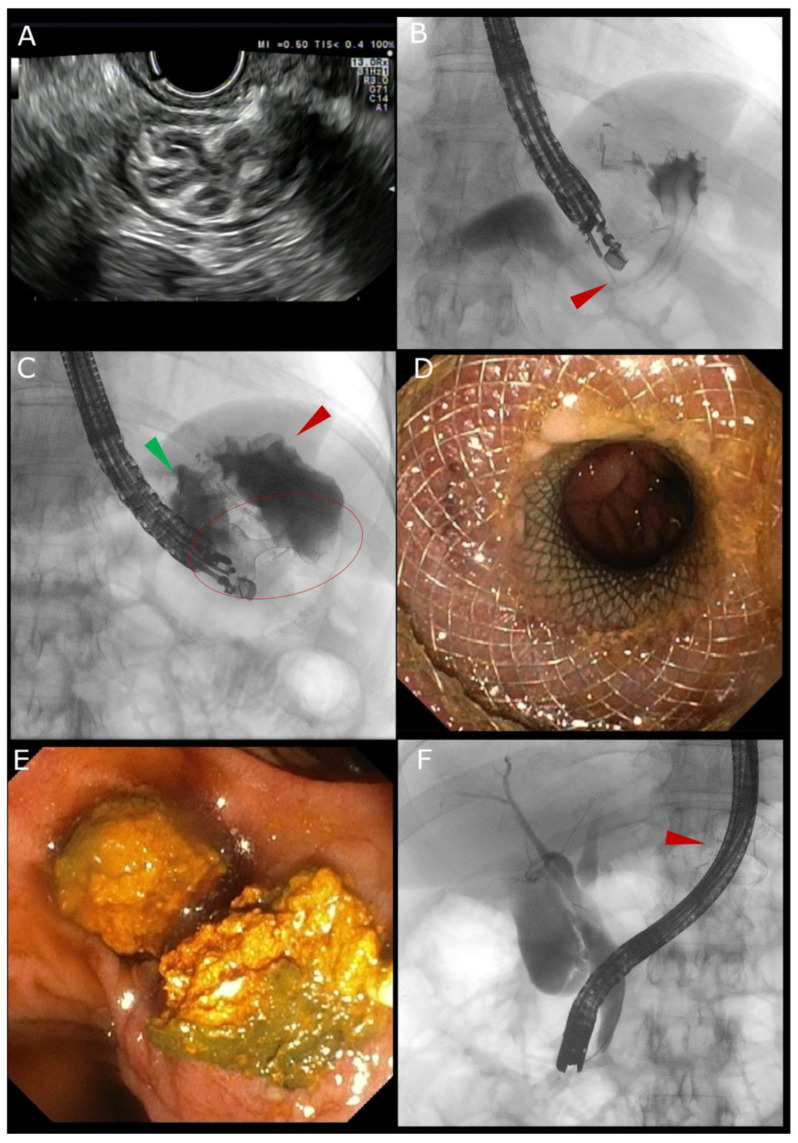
(A) Endoscopic ultrasound (EUS) view of the “sand dollar sign” for the identification of the excluded stomach. (B) EUS-guided contrast injection through the needle (red arrow) confirmed access to the gastric remnant by radiological view. (C) A 20 × 10 mm lumen-apposing metal stent (LAMS, red circle) was then placed between the gastric pouch (green arrow) and the excluded stomach (red arrow). (D) Endoscopic view of the excluded stomach through the LAMS. (E) Endoscopic view of the biliary stones extracted through conventional ERCP. (F) Radiologic view of the conventional ERCP conducted by passing a duodenoscope through the LAMS (red arrow).
